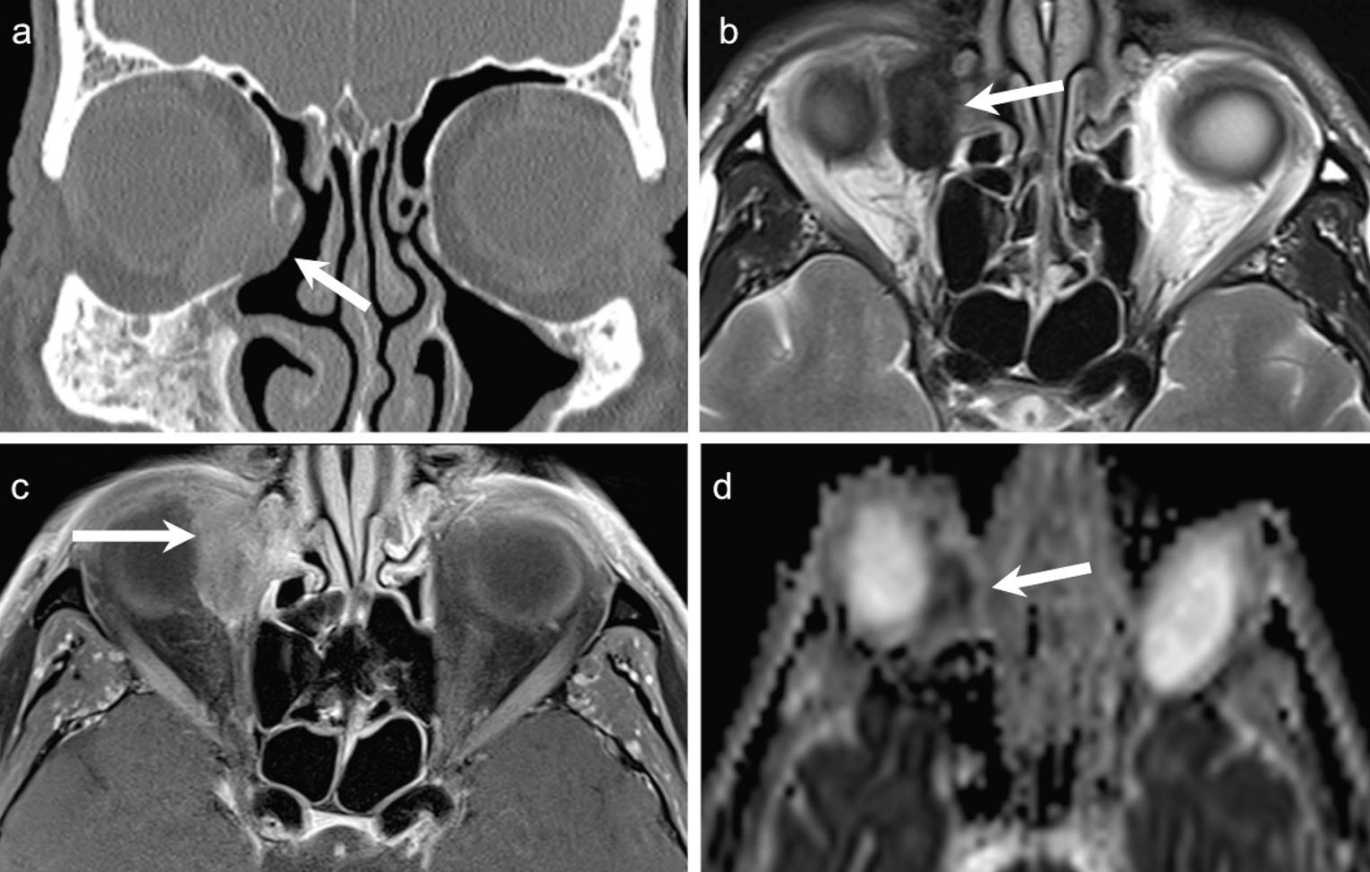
50-year-old male patient with biopsy-proven IgG4-RD of the right orbit. Coronal bone-window CT image of the orbits (a) shows a well-circumscribed, extraconal, soft tissue-density mass located inferomedially in the right orbit. It is associated with bony erosion of the lamina papyracea (arrow). b. Axial T2W image of the same patient shows very low signal within the well-circumscribed lesion (arrow). Note that it is lower than usually seen in lymphoma. c. Axial contrast-enhanced FS TIW image shows homogeneous non-specific enhancement within the lesion (arrow). d. ADC map shows restricted diffusion (ADC = 0.9 × 10 ^−3^ mm^2^/s)
